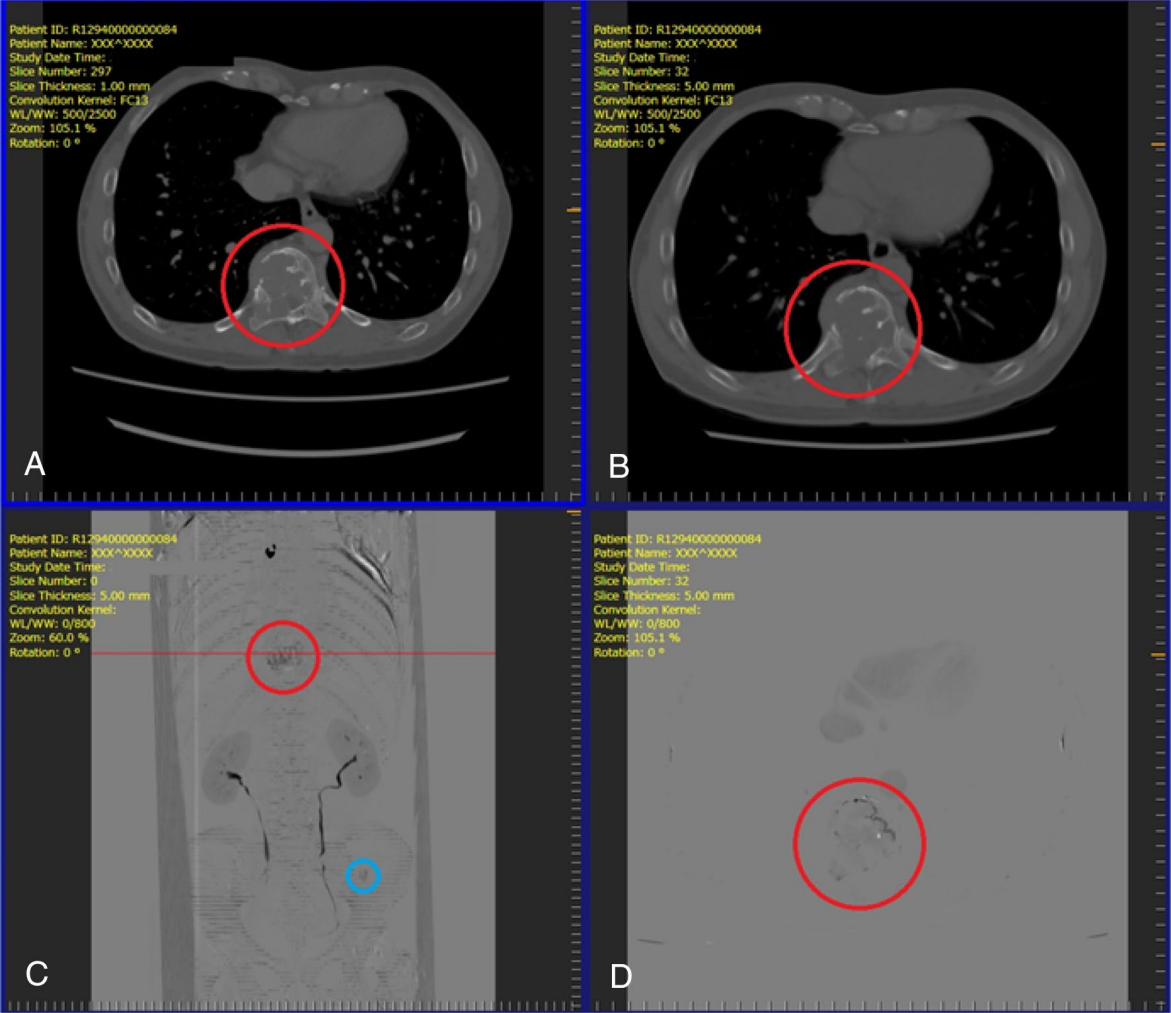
Screenshot of the image viewer for the observer study. (A) previous computed tomography (CT) image (upper left); (B) current CT image (upper right); (C) projection of temporal subtraction (TS) images (lower left); (D) TS image (lower right). When an observer clicks on a suspicious lesion, the dialog box appears to rate its likelihood (low to high) of being a bone metastasis. These representative images are obtained from a 55-year-old male patient with renal cell carcinoma who developed two osteolytic metastases in a thoracic vertebra (red circle) and the left iliac bone (blue circle). Both metastases are clearly visualized.
